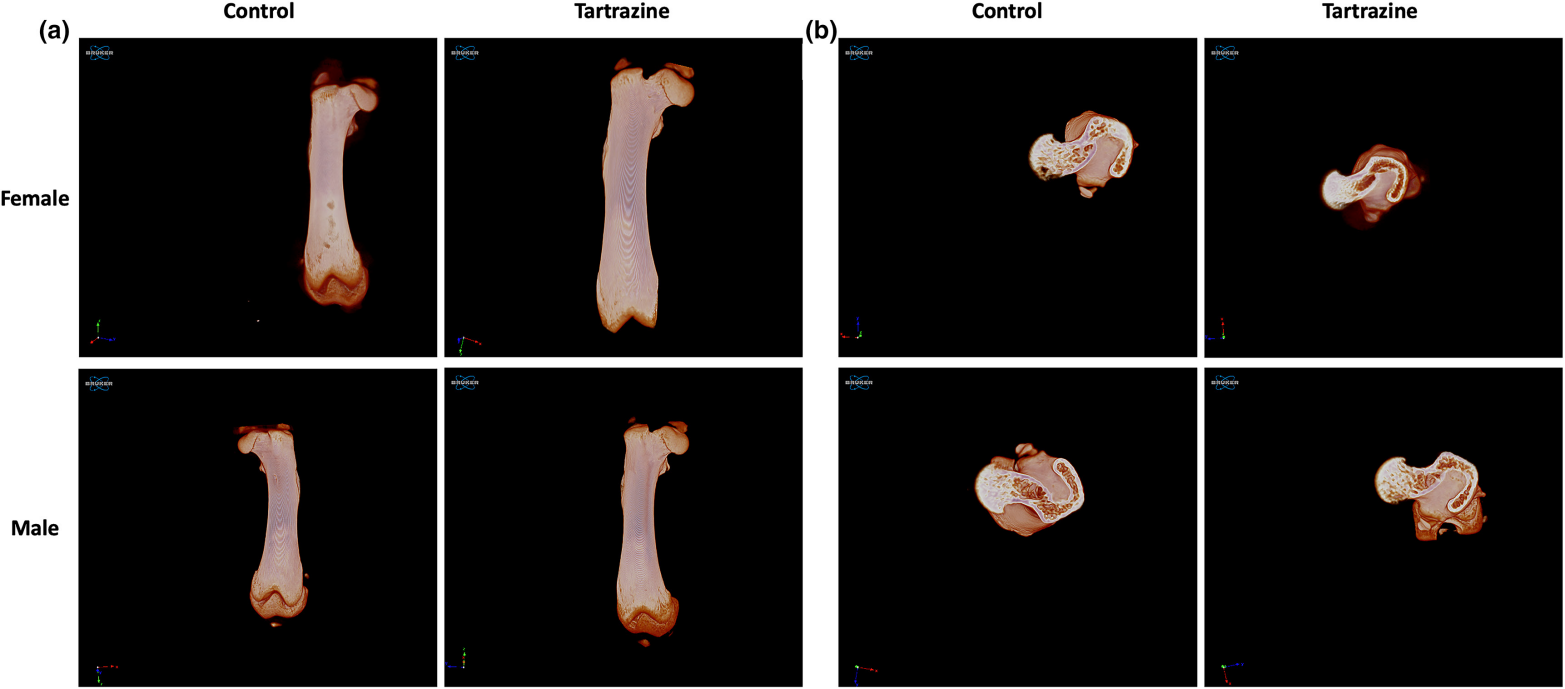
Micro‐CT scan of the femur (a), the micro‐CT images show that the trabeculae and trabecular separating head of the femur and greater trochanter can be seen in transverse section (b).
